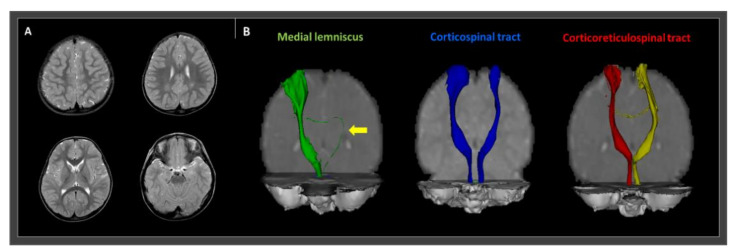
T2-weighted magnetic resonance imaging and diffusion tensor tractography (DTT) images of a 27-month old male cerebral palsied patient with gait instability. (A) T2-weighted magnetic resonance images show no abnormal lesions. (B) DTT revealing a thinned and disrupted left medial lemniscus (yellow arrow). However, the right medial lemniscus and both the corticospinal and corticoreticulospinal tract show preserved integrity to the cortex.
